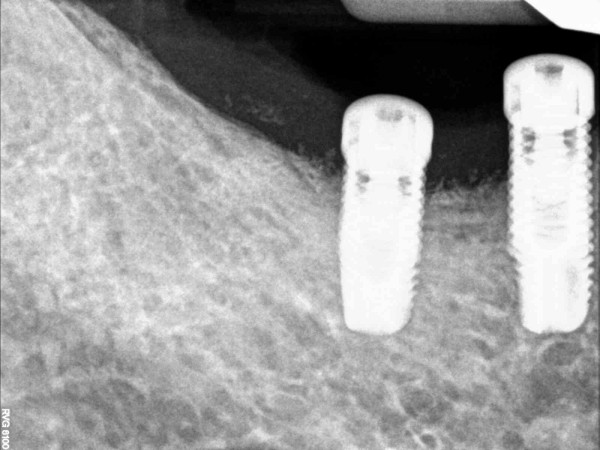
Postoperative radiograph 12 months after regenerative therapy.
